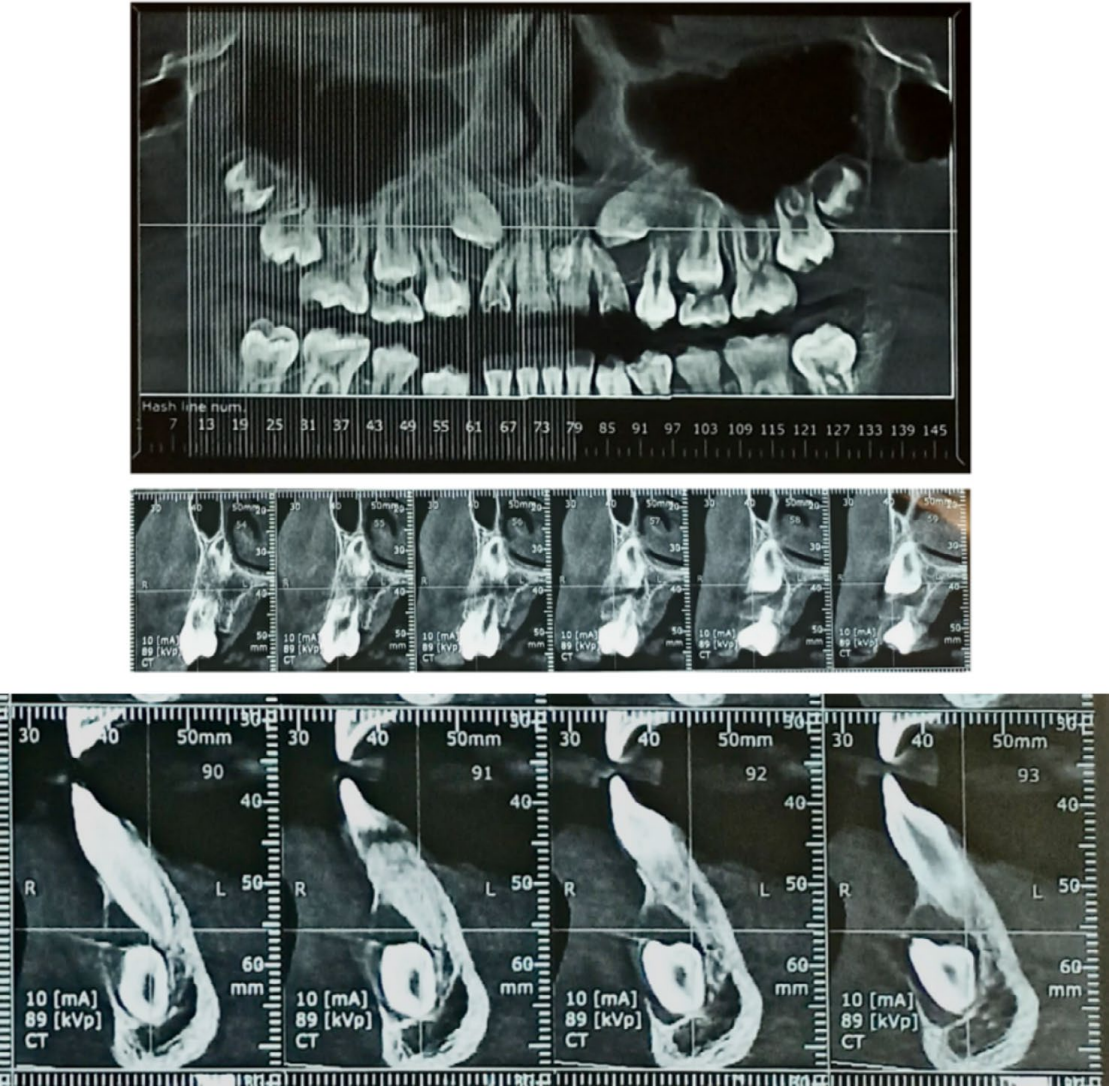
CBCT scans regarding root resorption in the first upper right bicuspid and erosion of the labial cortical concerning the cuspid.
